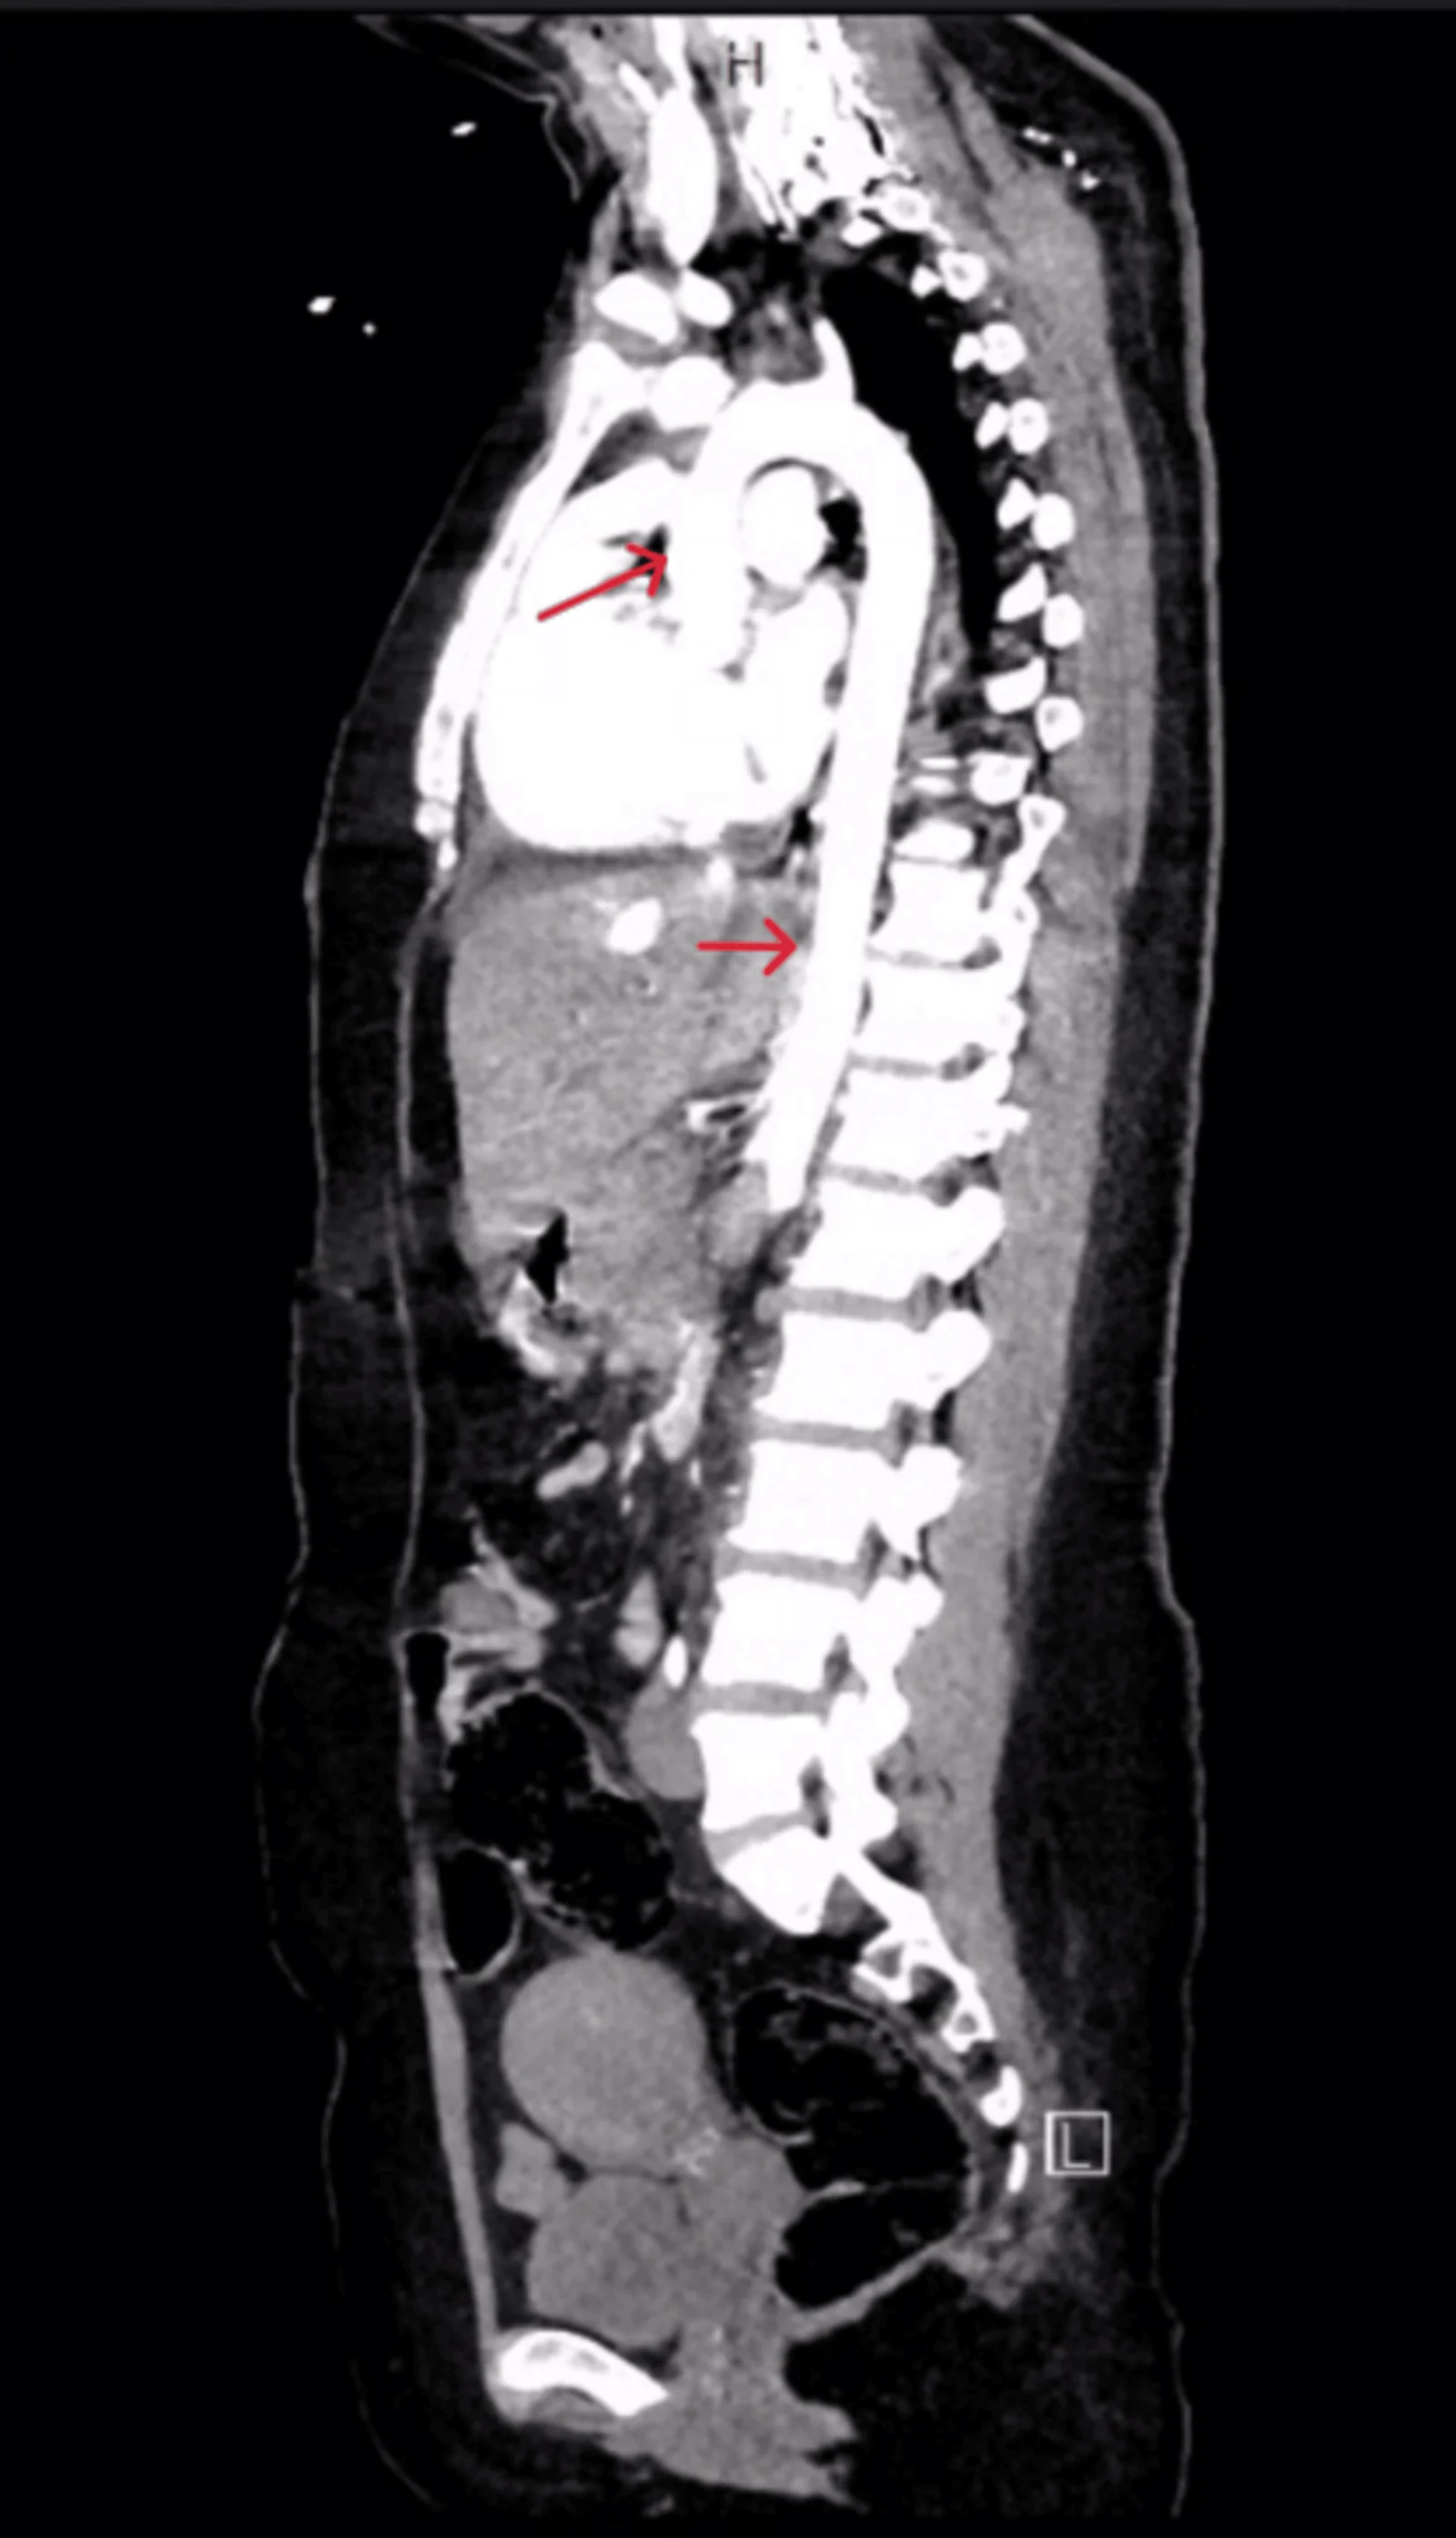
Computed tomography angiography (CTA) of the chest and abdomen demonstrating a patent and well-opacified thoracic aorta with contrast, with no aortic dissection, aneurysmal dilatation, significant stenosis, or occlusion (red arrows)
